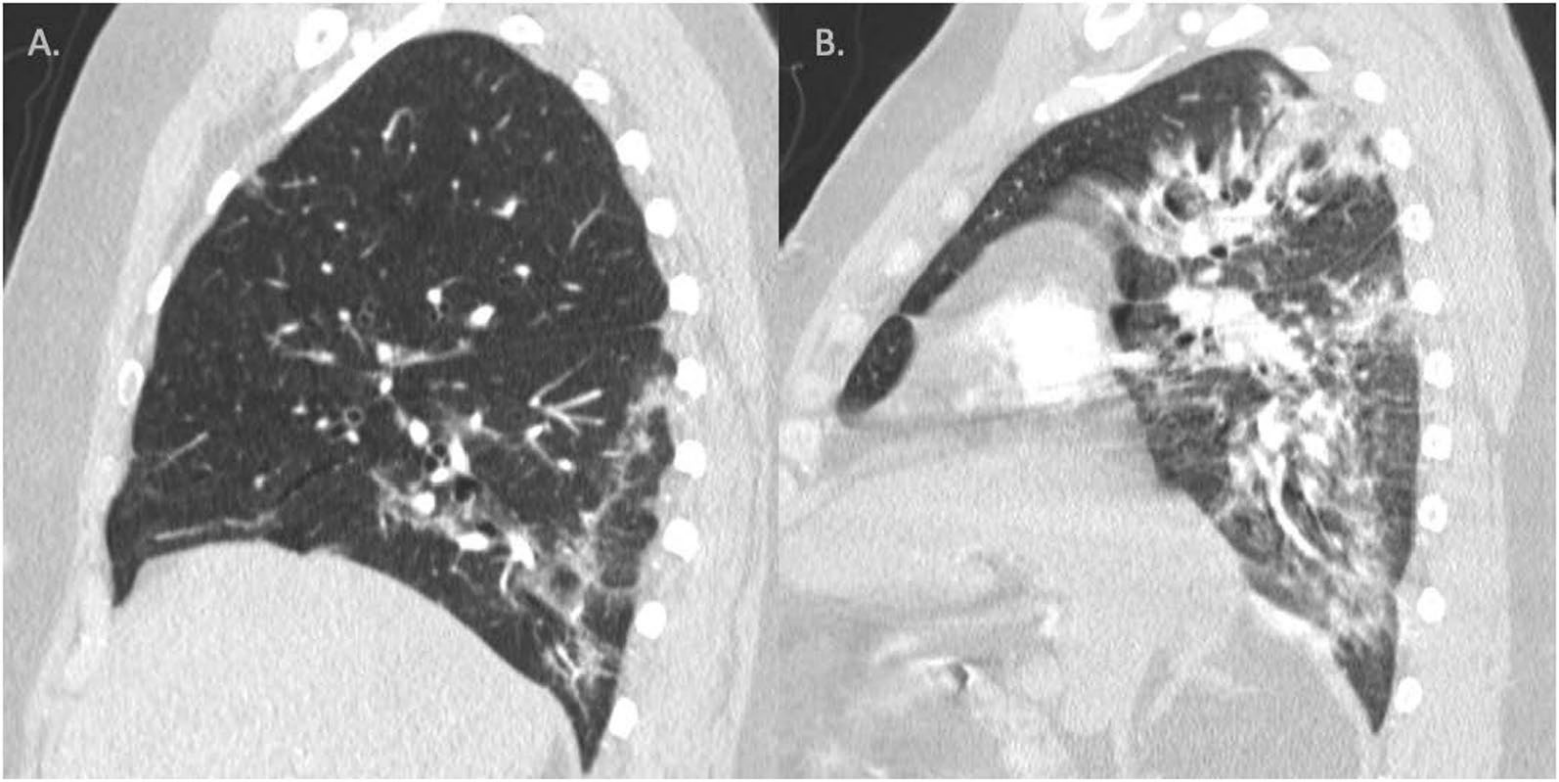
Representative images demonstrating the difference between a CT-SS scoring system. A Severity score = 1, B severity score = 2. *CT-SS* CT scoring system [26]
